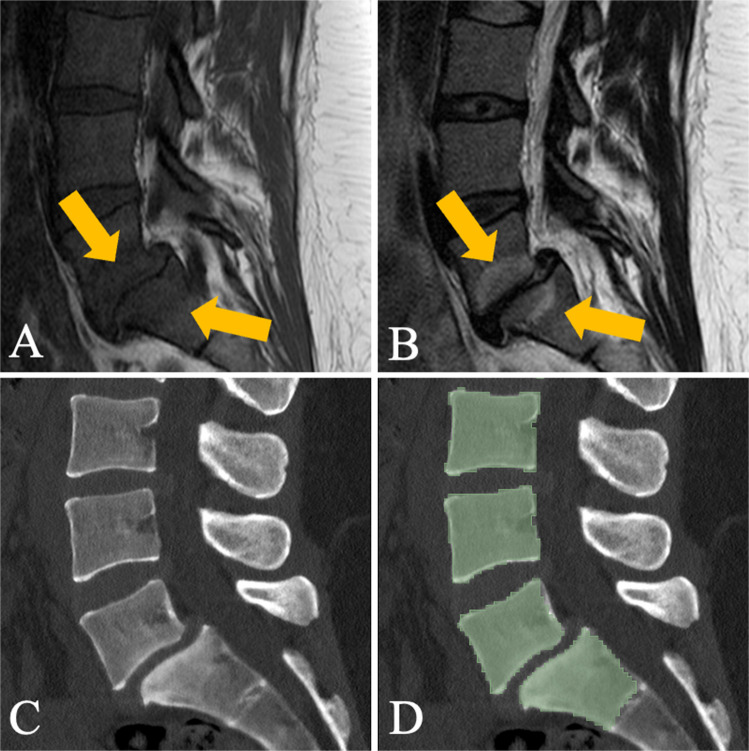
Representative sagittal T1-weighted (a) and T2-weighted MR image (b) of the lumbar spine of a 35-year-old female patient with bilateral spondylolysis and Modic type 1 changes of the L5/S1 segment (arrows). The subsequent photon-counting detector CT examination performed 5 days later is shown in (c). Overlaid masks of the vertebral bodies of L3-S1 for radiomics feature extraction are shown in (d). For this patient, the LASSO model estimated probabilities of Modic type 1 changes of 0.06 at L3, 0.08 at L4, 0.91 at L5, and 0.88 at S1
